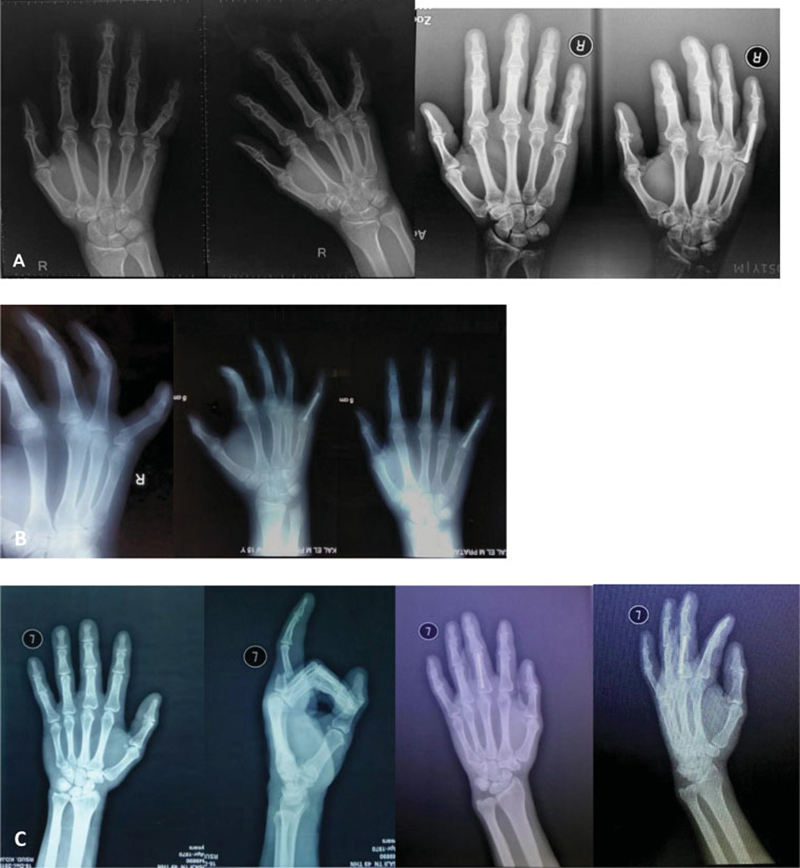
Preoperative (left) and postoperative (right) radiographic evaluations: (
